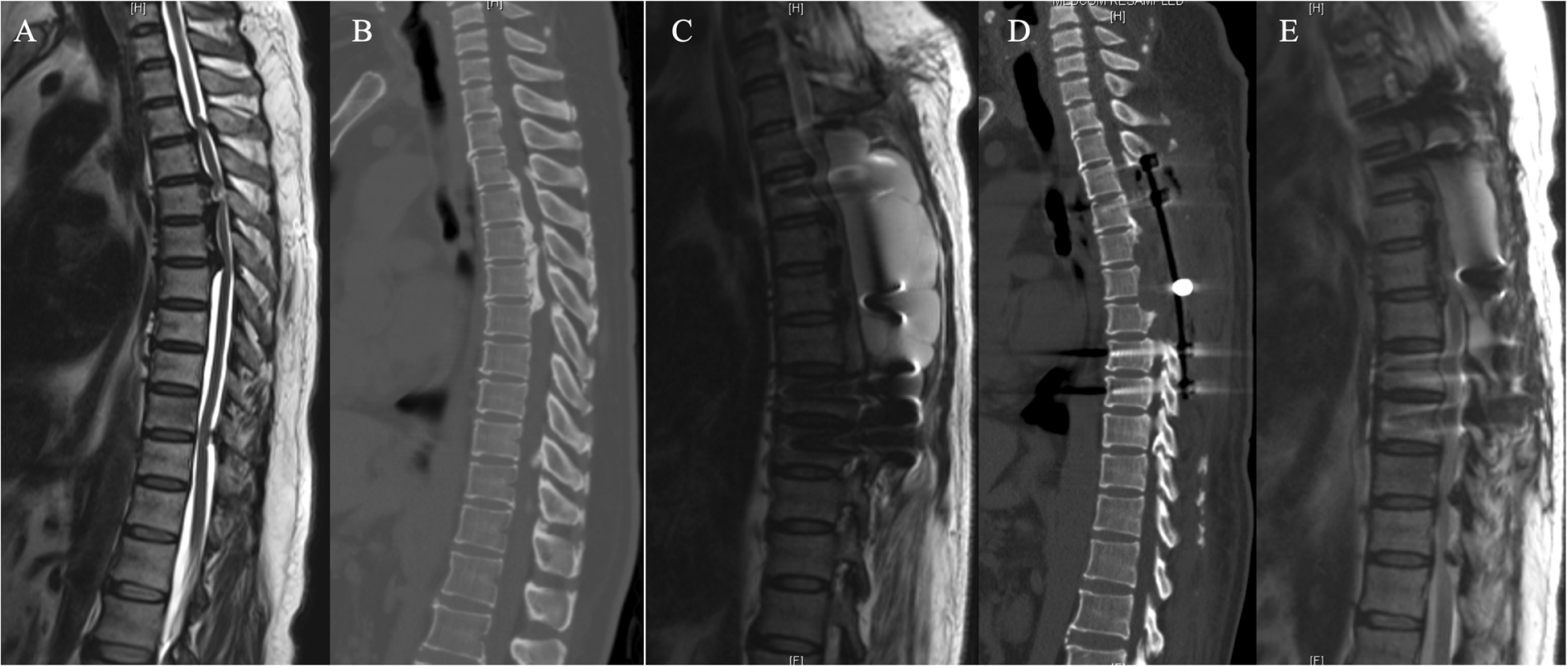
a, b Preoperative MRI and CT reveal that T4-8 OPLL and spinal cord compression. He received circumferential decompression from T4-8, with instrumentation from T3 to T9, intraoperative dural injury occurred, tight suturing of the muscle and fascia layers were implemented. Postoperatively, CSF was detected, and the wound drain was removed at 4th day. He discharged at 5th postoperative day. c, d Unplanned readmission occurred at 34th postoperative day due to the wound poor healing, MRI and CT reveal CSF around the decompression region. e He received revision surgery of tight suturing of the muscle and fascia layers, postoperative MRI revealed that the pseudomeningocele was cleared
